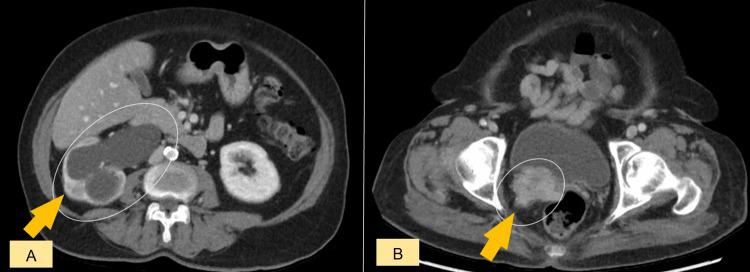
Contrast-enhanced CT scan of the chest, abdomen, and pelvis (axial views). (A) Right-sided hydronephrosis (arrow) caused by distal ureteric obstruction from the underlying mass. (B) Irregular mass (arrow) in the rectovesical pouch infiltrating the right posterior bladder wall and distal ureter, resulting in right hydroureteronephrosis.
